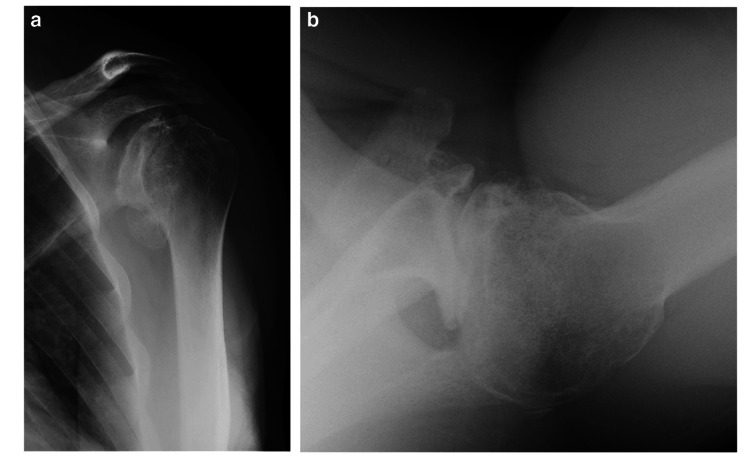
Preoperative Radiographs Demonstrating Glenohumeral Arthritis with Type B2 Glenoid Deformity Preoperative anteroposterior (AP) and axillary radiographs showing severe degenerative joint disease and a type B2 glenoid with biconcavity and posterior decentering of the humeral head. Used with permission from Matsen et al., International Orthopaedics (2019).
